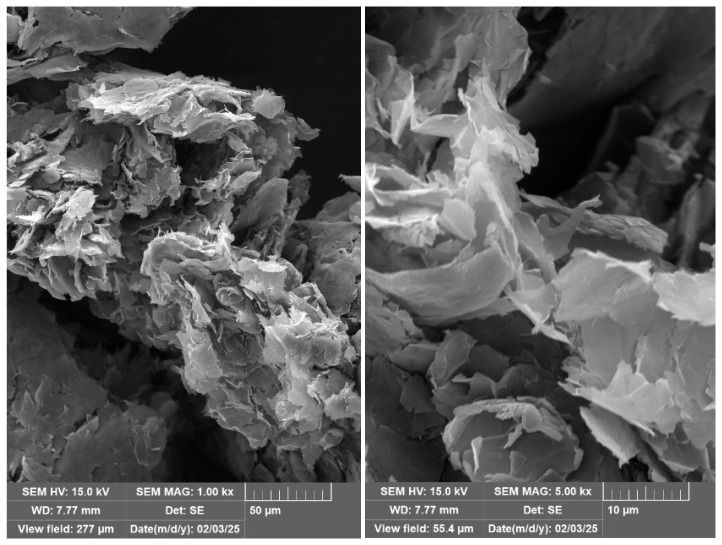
SEM of ultrasonicated expanded graphite at 1000× magnification (left) and 5000x magnification (right).
